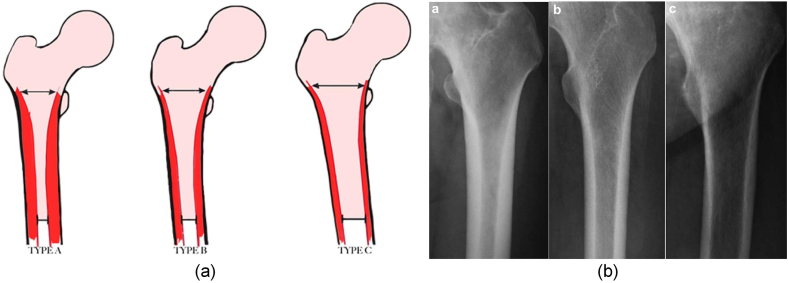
(a) Dorr classification [27] (b) Radiography of preoperative proximal femoral geometry categorized using the classification proposed by Dorr [28].
